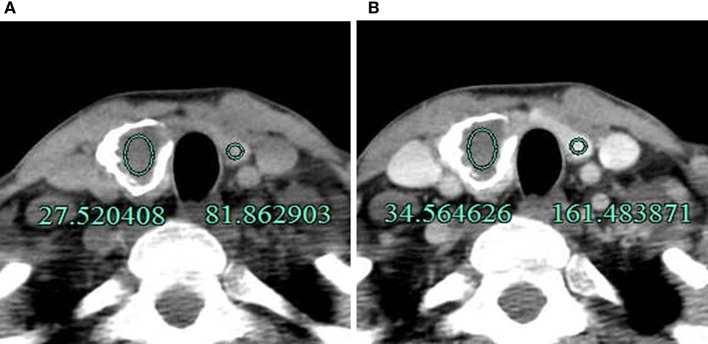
A 40-year-old man with an annular calcified nodule on the right lobe of the thyroid. (A) The difference in CT value between the thyroid tissue and inside the calcified nodule was 54.34 Hu (81.86–27.52 Hu) on unenhanced CT. (B) The difference after enhancement was 126.92 Hu (161.48–34.56 Hu) and was higher compared to unenhanced CT. The lesion was pathologically confirmed as NG.
